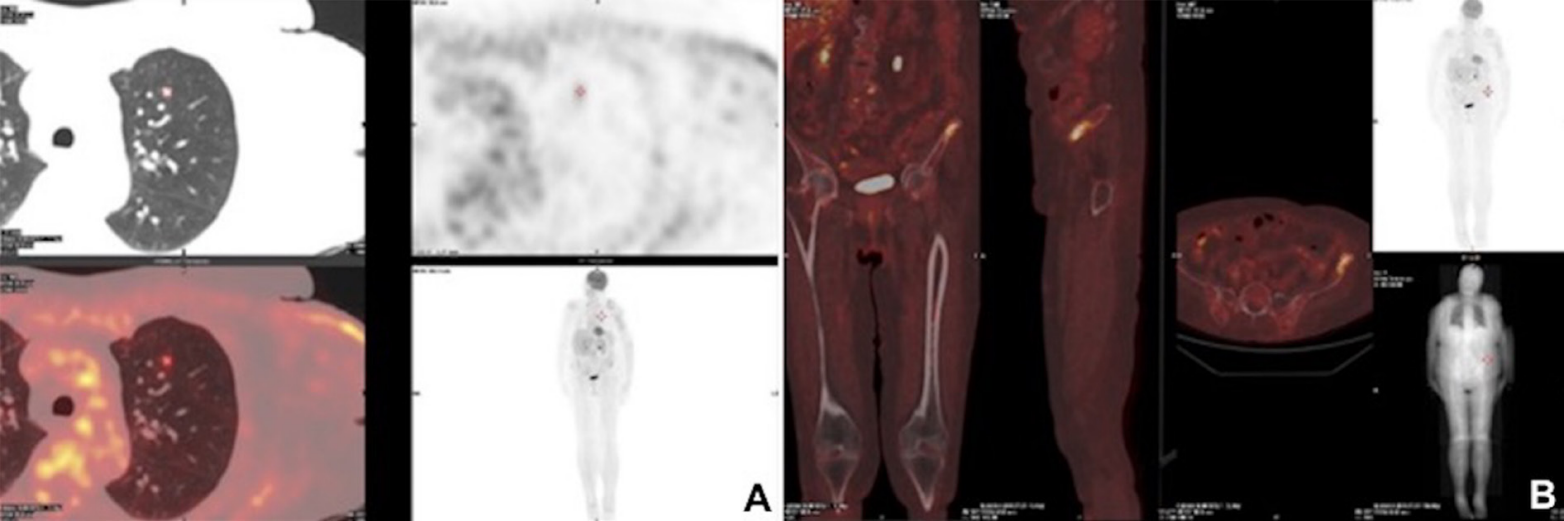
Postoperative radiological images. Three-months postoperative PET/CT scan showing a FDG-avid, presumptive pathological uptake in left lung (SUV max = 2.0) (A) and in left iliac wing (SUV max = 7) (B).
